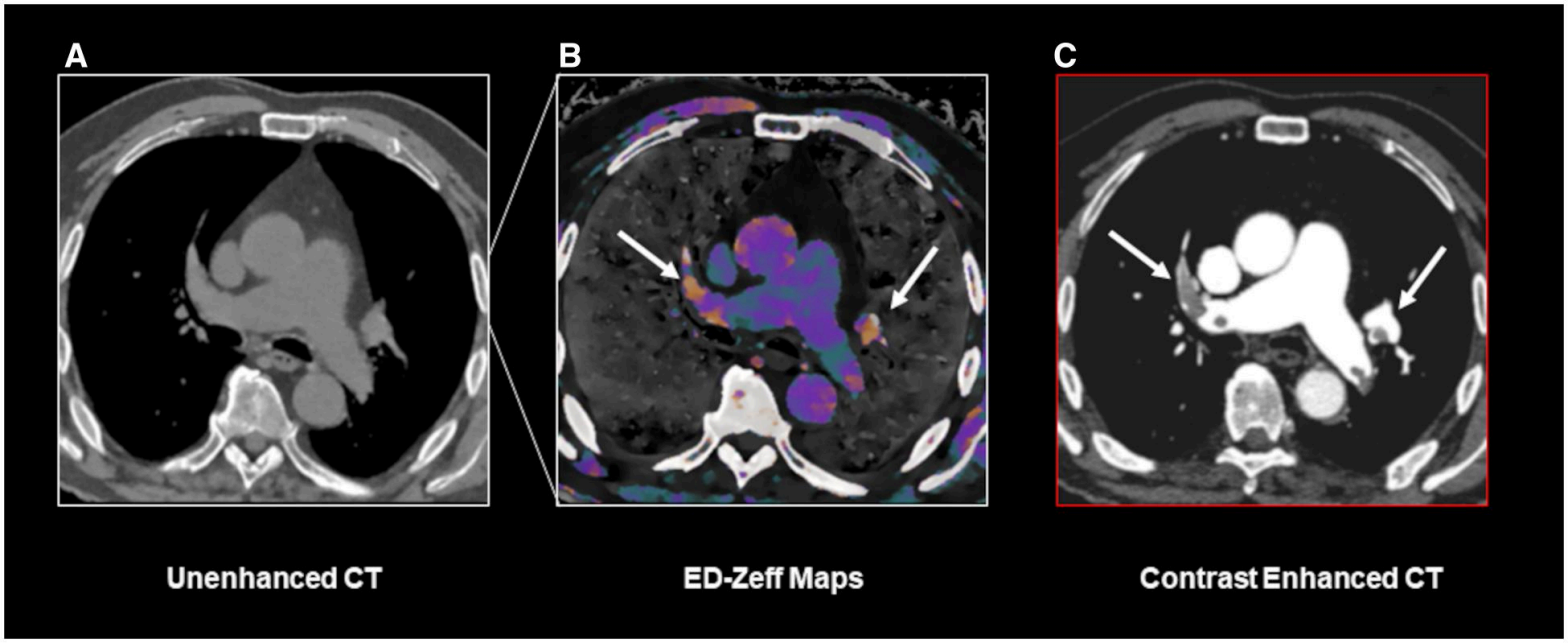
A true positive case of PE identified using the ED-Zeff (electron density-Z-effective) map. An 83-year-old male patient presenting to our department with dyspnea, tachypnea, and chest pain. Unenhanced CT scan of the chest (A) shows only mild dilatation of main pulmonary artery. ED-Zeff map (B), derived from the unenhanced CT scan, shows the presence of high signal spots (arrows) within the right and left pulmonary arteries (arrows). The subsequently performed CECT (contrast-enhanced computed tomography) (C) confirms the presence of pulmonary emboli (arrows) at the same location as shown in B.
